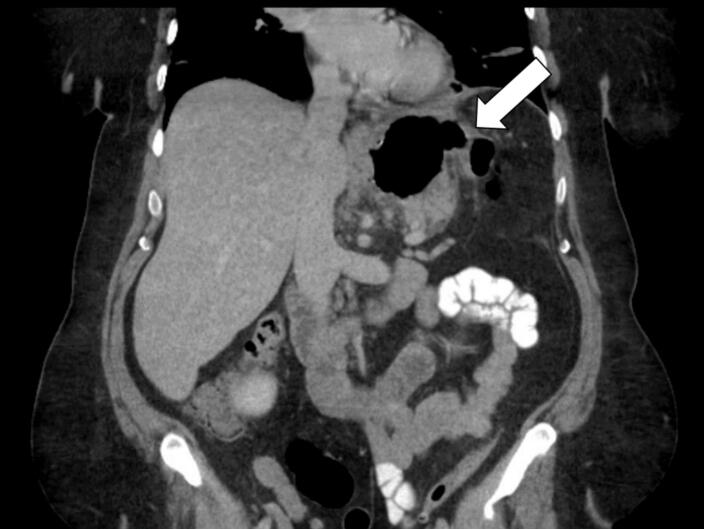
A computed tomography showing the cavity and an early gastrocolic fistula.
